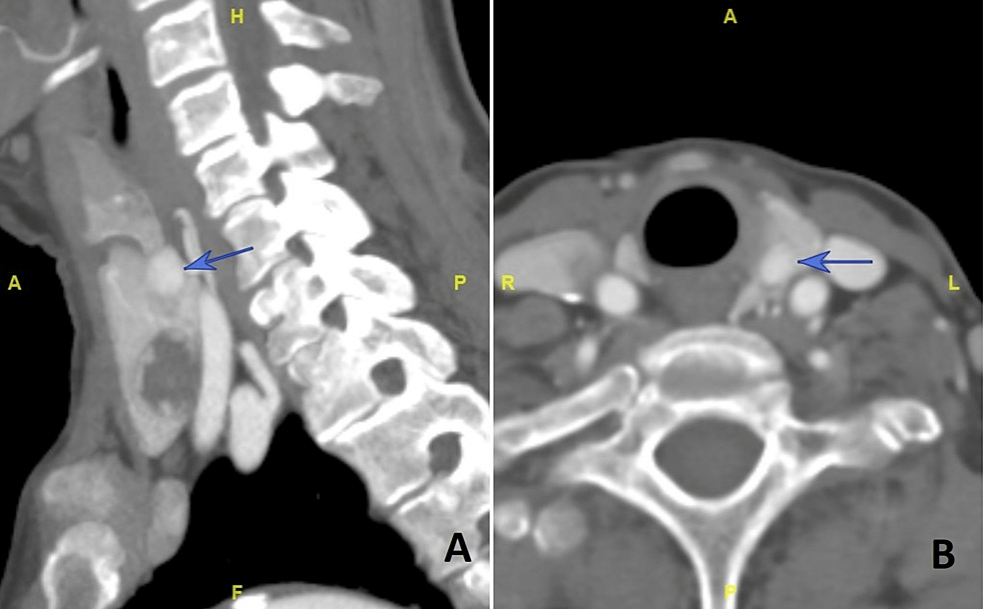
Computed tomography, sagittal (A) and axial (B) imaging, in the arterial phase following the administration of intravenous contrast medium, demonstrated a well-circumscribed arterially enhancing lesion (blue arrow) posterior and medial to the left lobe of the thyroid.
